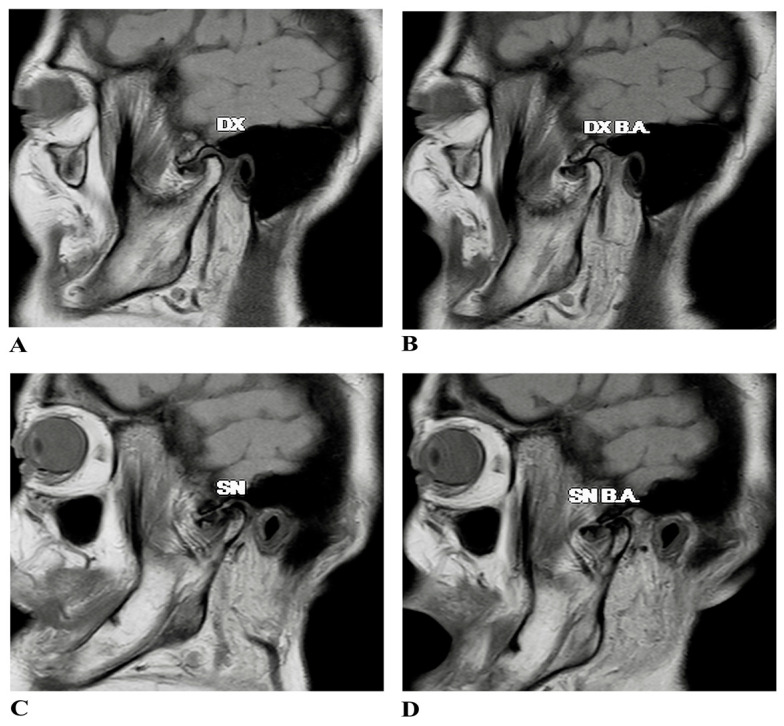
Proton density (PD)-weighted magnetic resonance imaging (MRI) of the right and left TMJs in closed and open mouth positions. (A–D) The MRIs of both TMJs show anterior disc dislocation without reduction. (A,C) In the closed position (maximum intercuspal), the intermediate zone of the disc is situated anterior to the condylar head, with the posterior part located anterior to the 11:30 position. (B,D) The intermediate zone maintains its anterior position relative to the condylar head in the open position.
